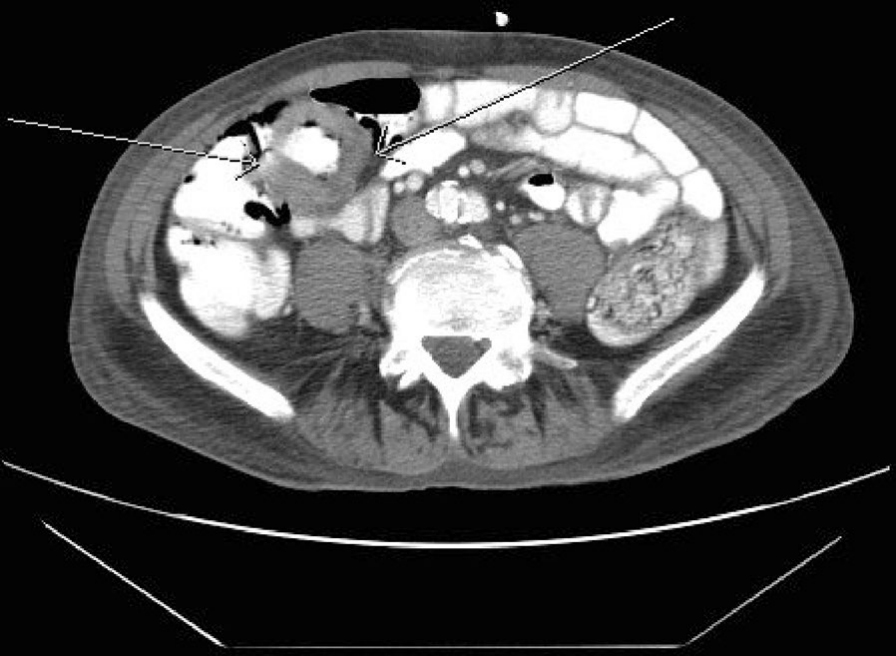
A mass at the ascending colon demonstrated on CT scan of abdomen and pelvis. The *arrow* showed a circumferential thickening of a segment of ascending colon due to colonic mass
